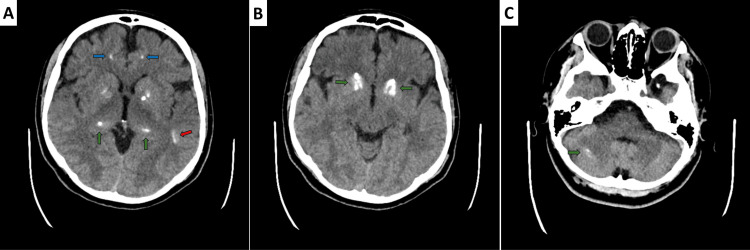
Non-contrast CT of the head, axial view, showing extensive brain calcifications (A) The blue arrow indicates the calcification in the subcortical white matter of the frontal lobe; the green arrows highlight the bilateral calcification in the thalami; and the red arrow points to the calcification in the temporal lobe subcortical white matter. (B) The dense, bilateral calcification within the basal ganglia. (C) The calcification within the cerebellum. CT: computed tomography
